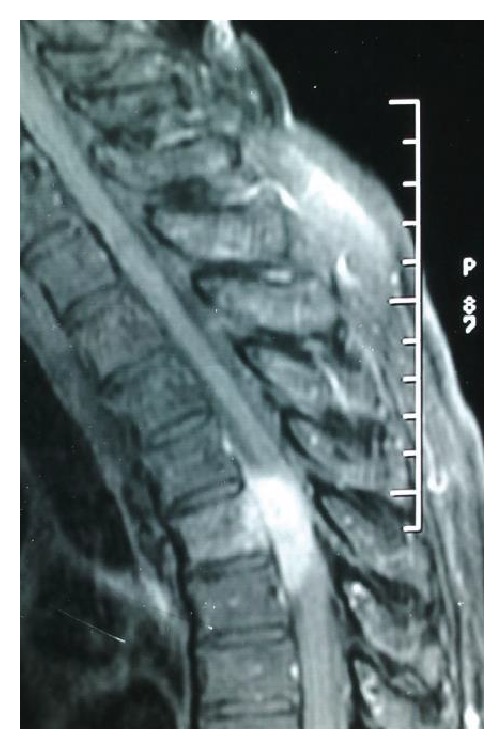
Preoperative sagittal T1-weighted postgadolinium MR image showing important tumor enhancement.
